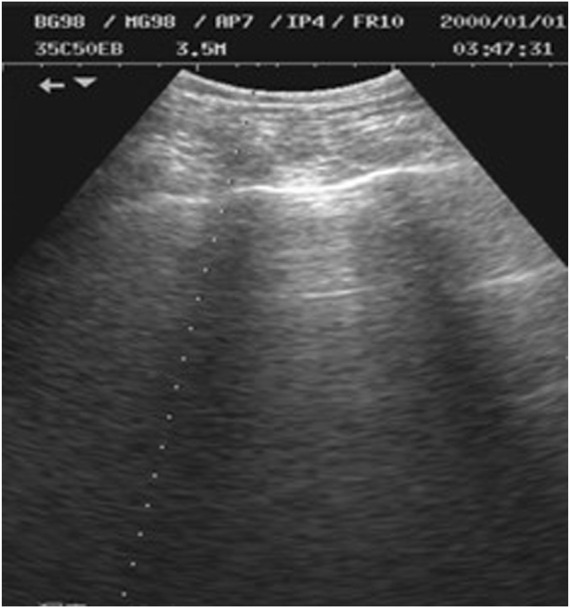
B-mode ultrasound rib image.
